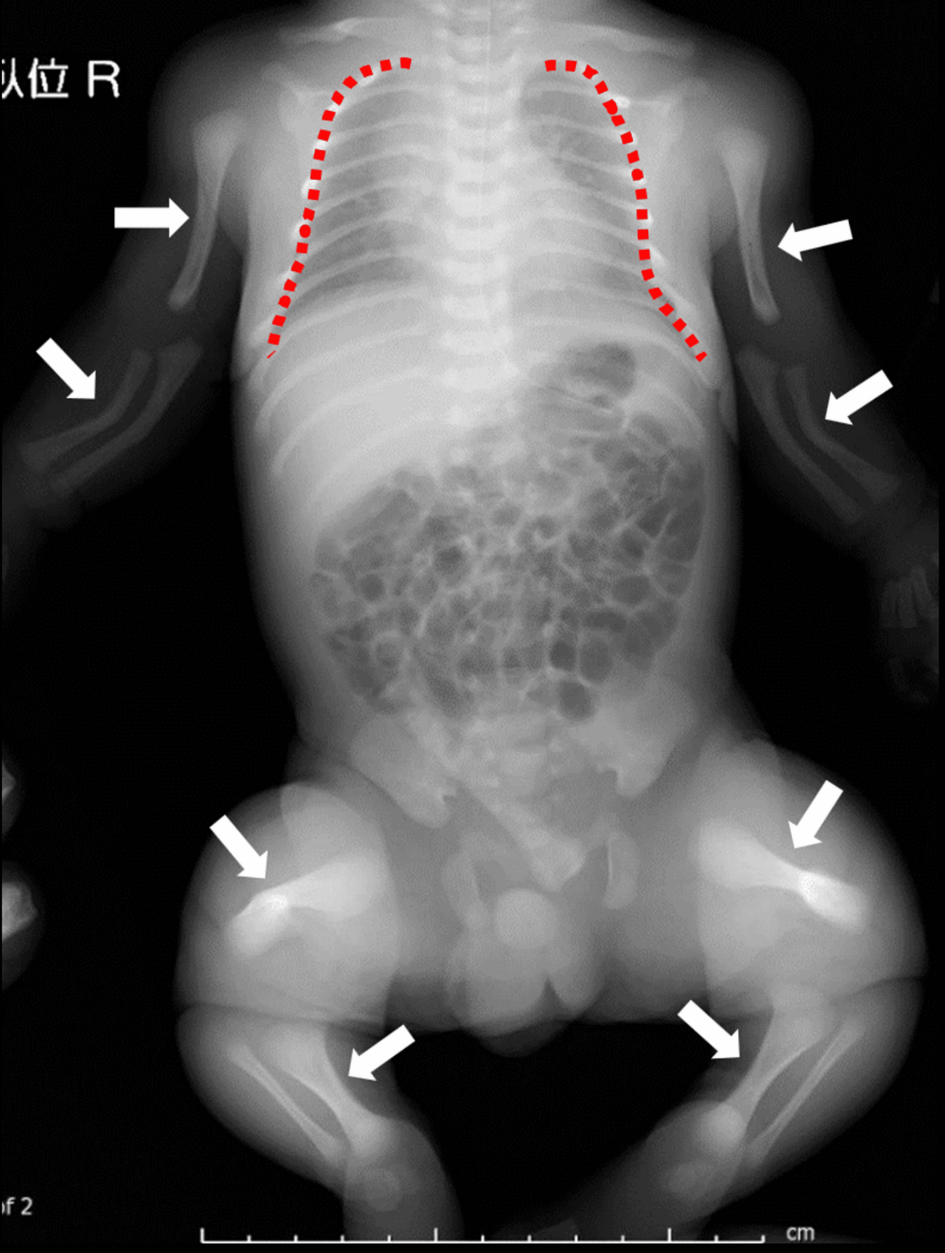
X-ray of the neonate's trunk Humerus, forearm, femur, and lower leg bone kyphosis were noted (white arrow) with a small thorax (red dotted line).
